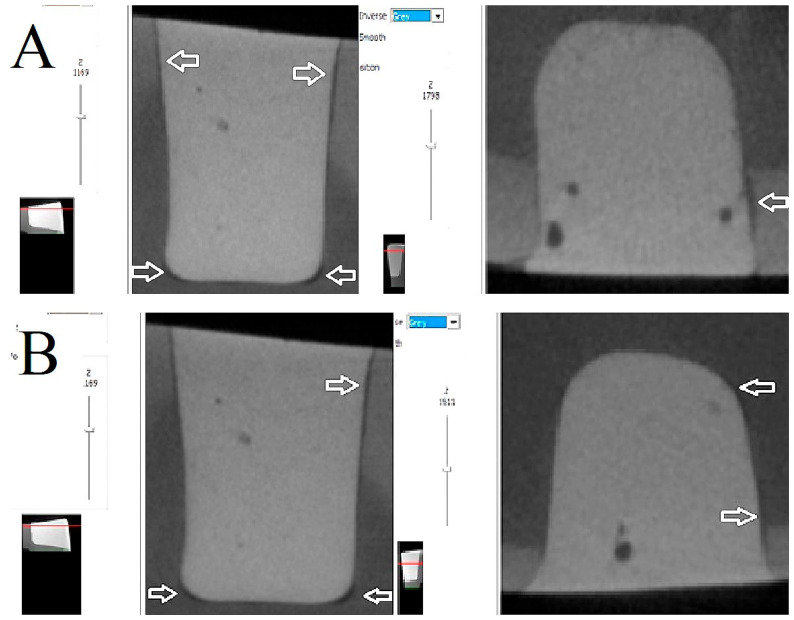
Representative images of microcomputed tomography analysis of Filtek Z250 layered restorations bonded with self-etch (A) and total-etch (B) adhesives. The presence of white arrows denotes the location of the internal gap.
